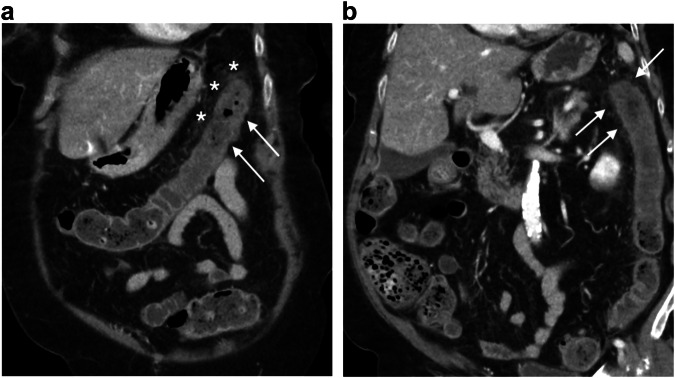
Ischemic colitis. Axial and coronal portal phase CT (a, b) in a 90-year-old man shows a segmental thickening of the left colonic angle with a stratified enhanced wall (arrows) and peripheric fat stranding (asterisk), suggesting a reperfusion form of ischemic colitis
